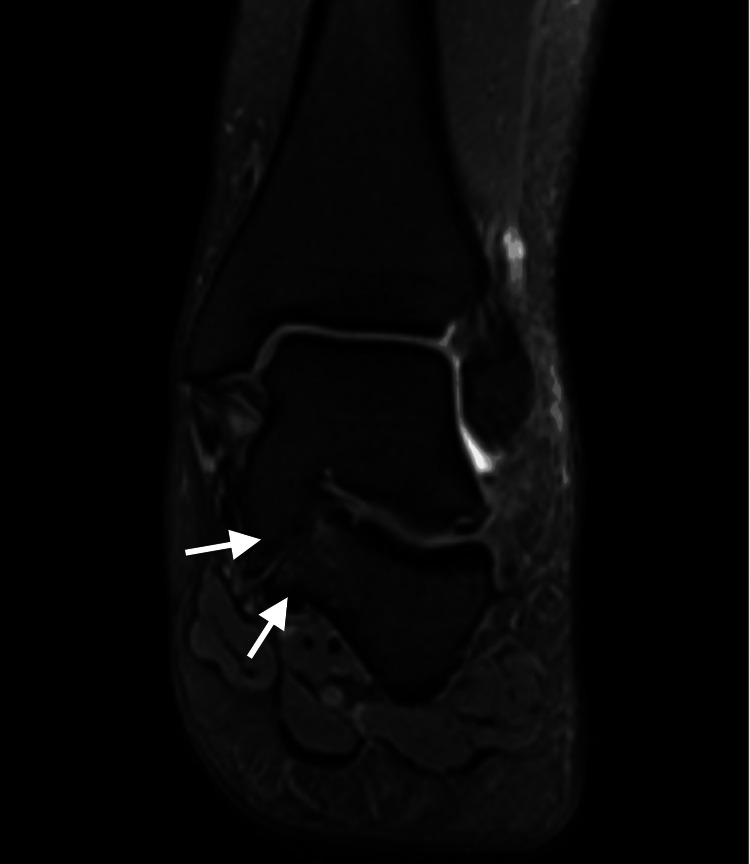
Coronal MRI demonstrating a type 3 shingled talocalcaneal coalition with fusion of the sustentaculum tali to the talus MRI: magnetic resonance imaging
